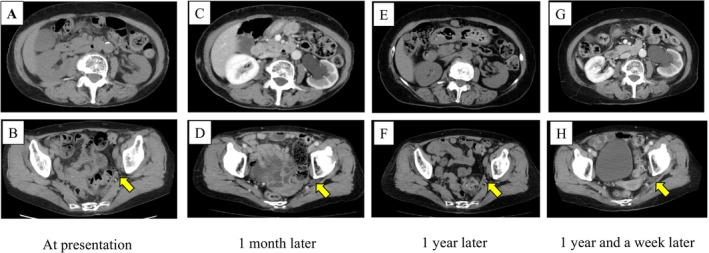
Spontaneous resolution and intermittent recurrence of USH. (A–H) CT images at four different time points: At presentation (A, B), 1 month later (C, D), 1 year later (E, F), and 1 year and 1 week later (G, H). The upper panels show axial images at the kidney level, and the lower panels show axial images at the pelvic level. The yellow arrows indicate the location of the left ureter.
